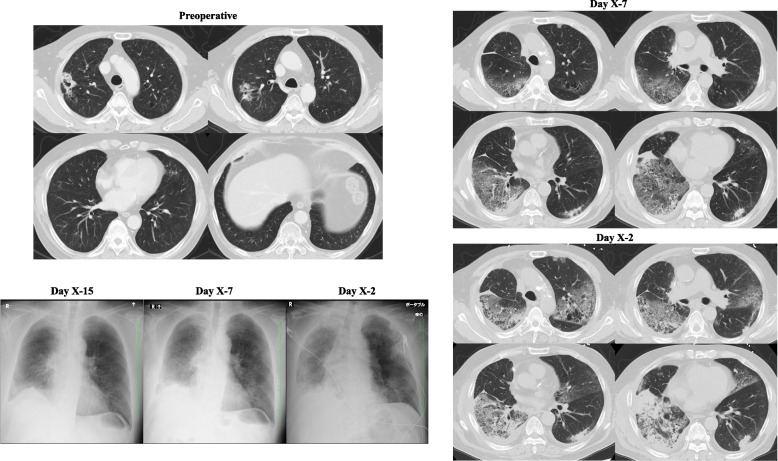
X-ray and computed tomography images of the patient’s chest before and after lung cancer resection. Preoperative examination revealed a lung cancer lesion in the right upper lobe. Examination upon postoperative readmission showed diffuse frosted shadows predominantly in the right lung (day X-7). However, upon intensive care unit (ICU) admission (day X-2), there were enlarged diffuse frosted shadows in both lung fields
